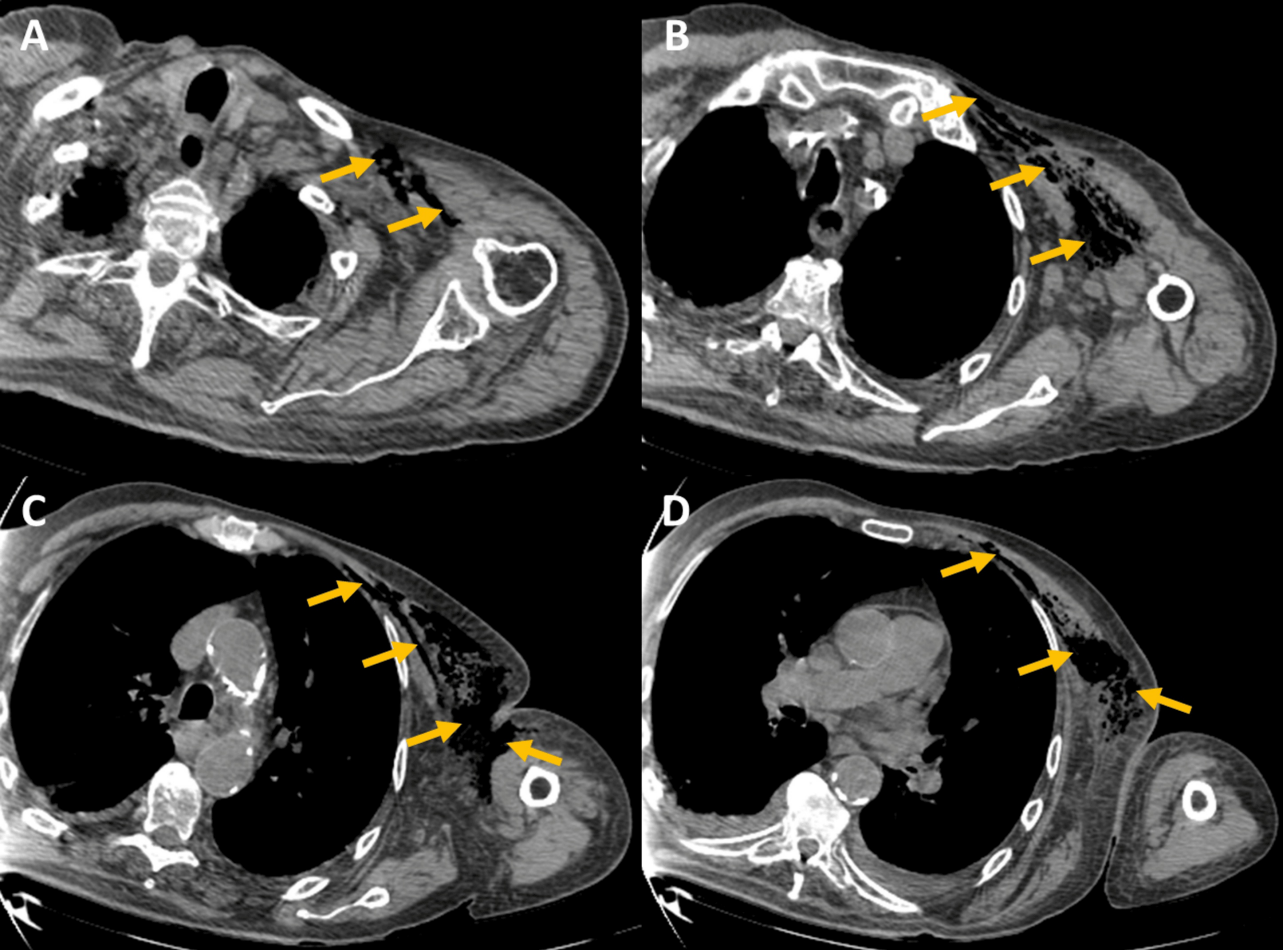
Chest and left upper limb CT scan, shown in multiple axial planes (A-D), revealing gas collections within the soft tissues (yellow arrows) and extensive densification of the subcutaneous fat in the left anterior chest wall and left upper limb.
